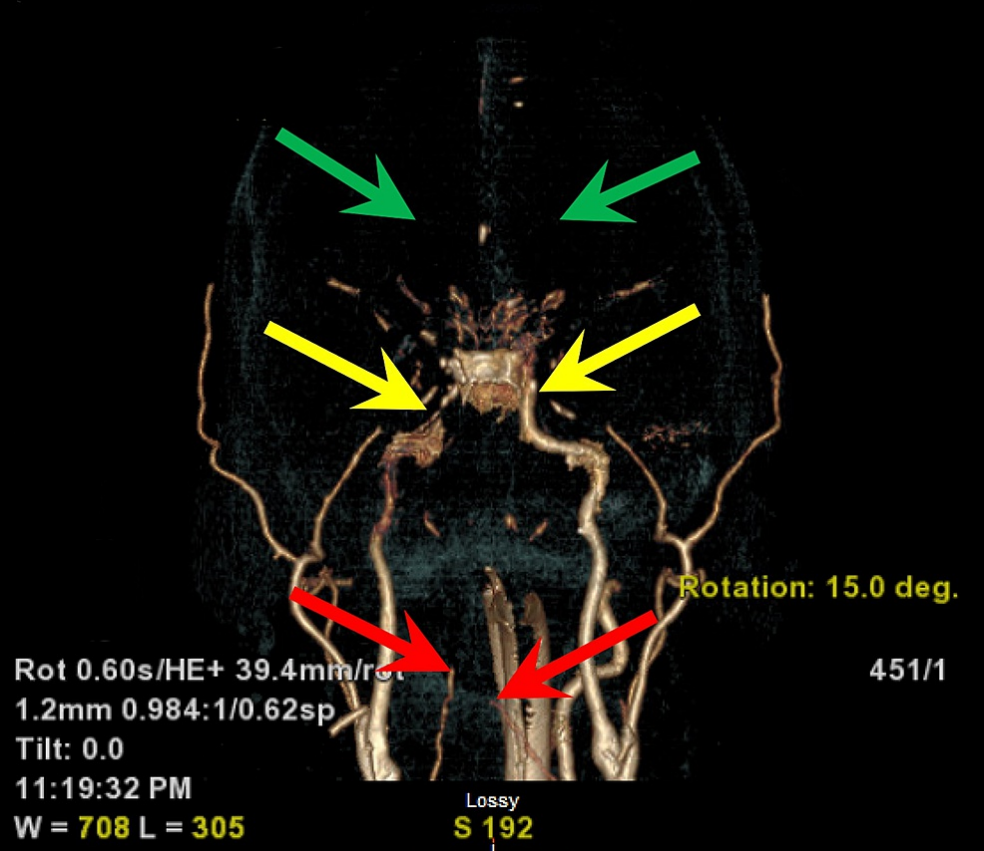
Reconstructed image of the CTA head and neck shows complete occlusion of bilateral vertebral arteries (red arrows) and complete occlusion of bilateral internal carotid arteries (yellow arrows) along with lack of intracranial circulation (green arrows) CTA, computerized tomography angiography
